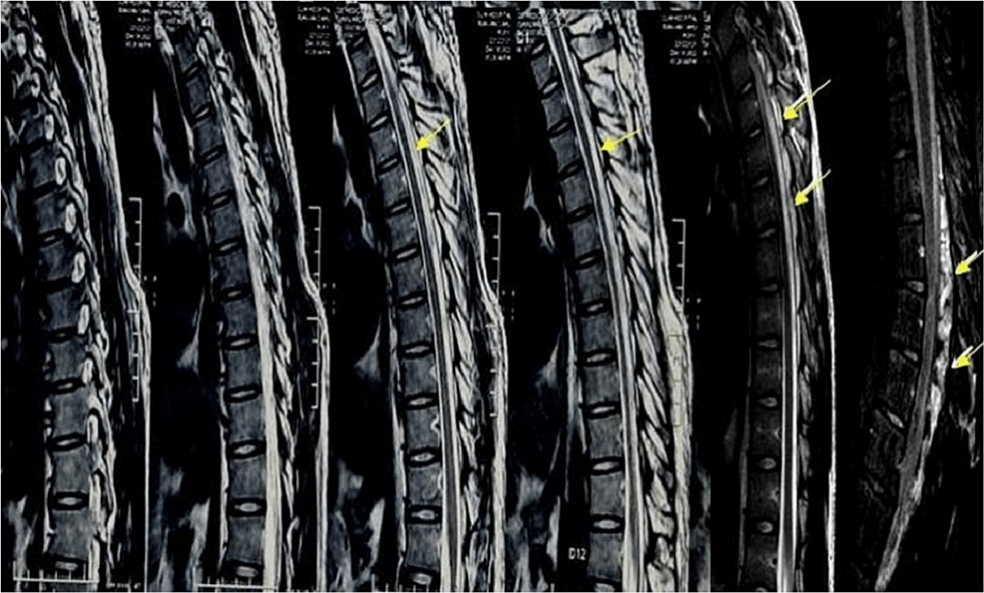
Flexion MRI sequence of thoracic spine showing increased posterior epidural space, further confirmed by contrast MRI showing increased venous prominence and posterior void (yellow arrows).
